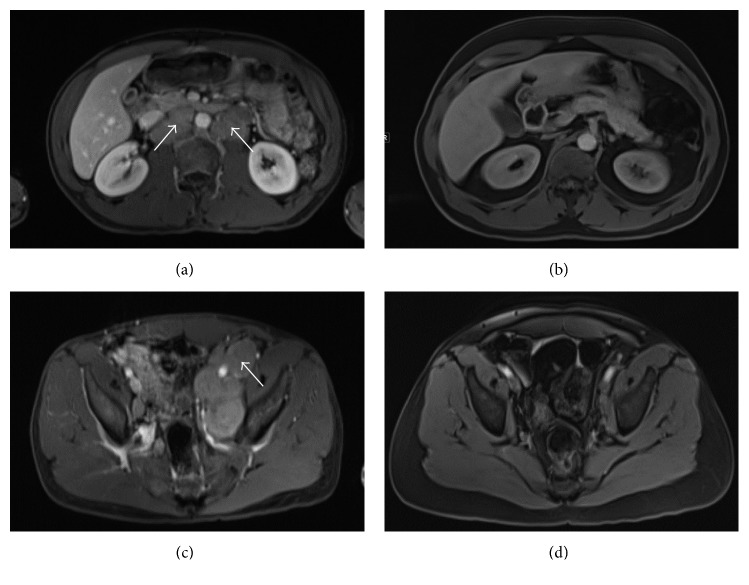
MRI of the patient's abdomen and pelvis showing resolution of paraaortic and iliac lymphadenopathy after ADT and docetaxel treatment. (a) Paraaortic lymphadenopathy (shown by white arrows) before treatment. (b) Resolution of paraaortic lymphadenopathy after treatment. (c) Left iliac lymphadenopathy (shown by white arrow) before treatment. (d) Resolution of left iliac lymphadenopathy after treatment.
